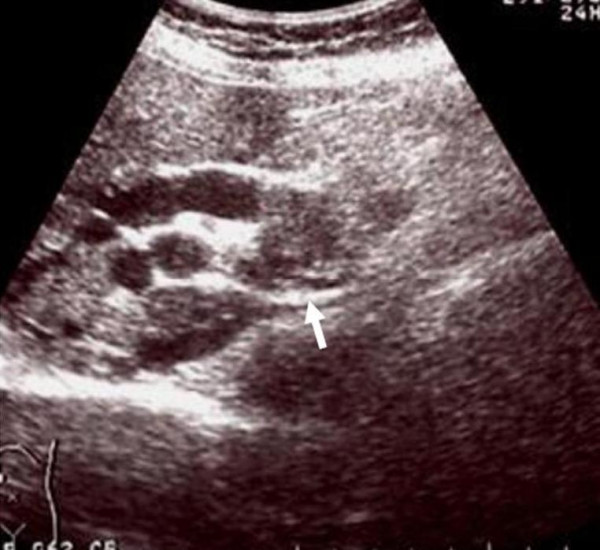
Intraoperative ultrasound clearly demonstrates the portal vein tumor thrombus.
